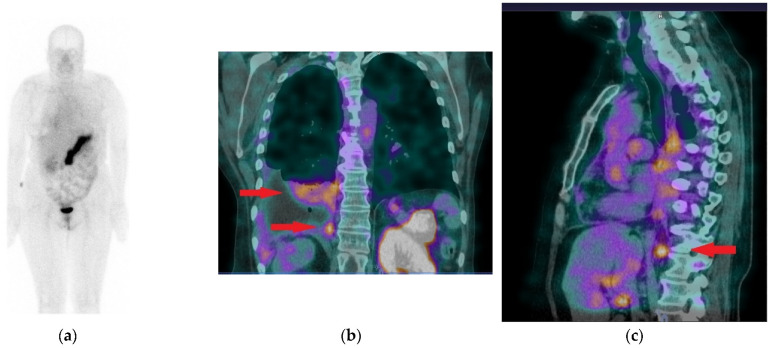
(a) Whole body scan of Patient 2, obtained 3 h after injection of [^99m^Tc]Tc-DB15 in the anterior projection shows physiological accumulation in the pancreas and increased uptake in the right pleura. (b) SPECT/CT coronal image of the chest presenting increased tracer uptake in the metastatic infiltrations in the pleura and lung parenchyma (red arrows). (c) SPECT/CT sagittal image of the chest depicting increased radioactivity accumulation in an enlarged (metastatic) phrenic lymph node (red arrow).
